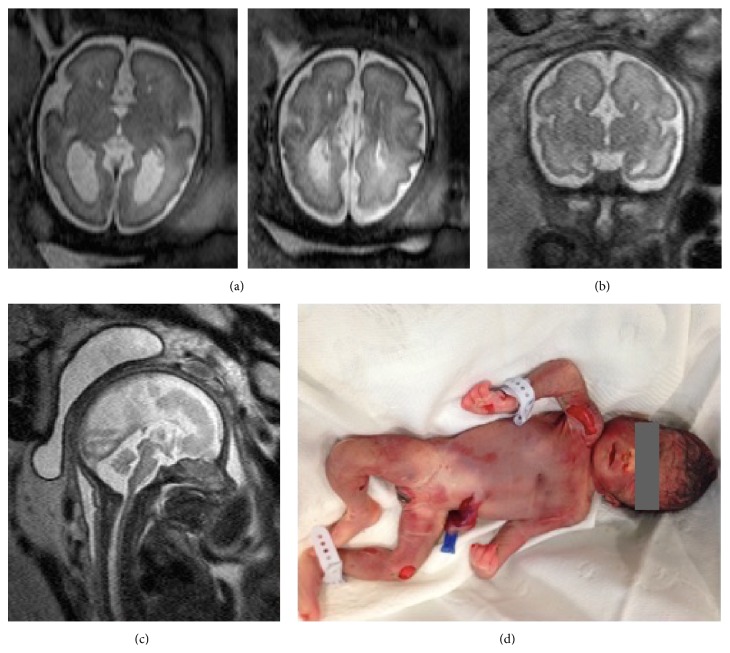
Fetal MRI images of absent corpus callosum and the picture of the newborn. (a) Axial scans at the level of the atria and bodies of the lateral ventricles show bilateral posterior ventriculomegaly. Anteriorly the lateral ventricles are narrow and parallel. This is the appearance of colpocephaly which is characteristically associated with ACC. (b) Coronal MRI scan at the level of third ventricle shows agenesis of the corpus callosum. The bodies of the lateral ventricles have a lateral convexity in the coronal plane and a medial concavity due to the presence of Probst's white matter bundles. This is the typical appearance in callosal agenesis. (c) Midsagittal MRI scan confirms absence of the corpus callosum. (d) Photograph taken in the postnatal period.
